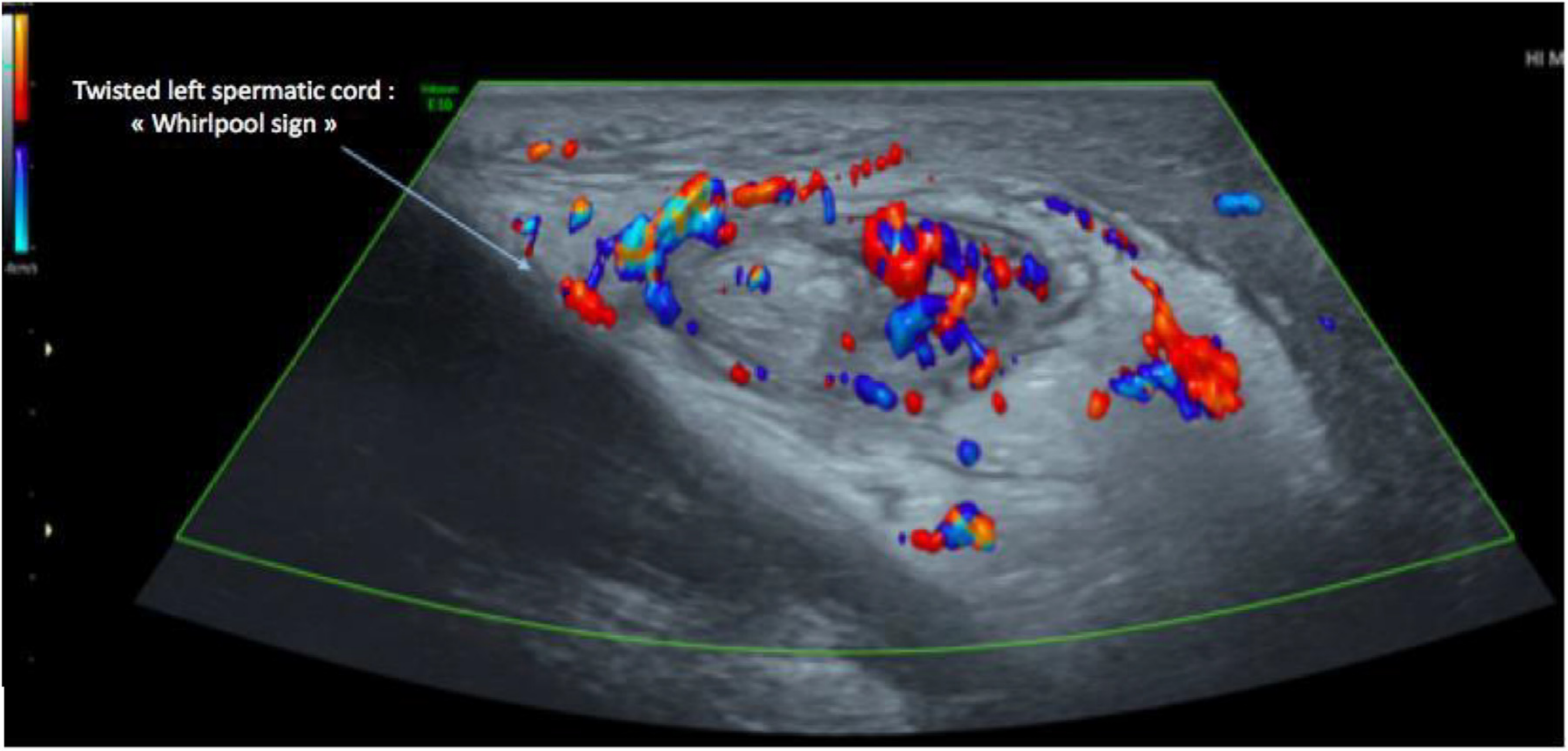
Study of the left spermatic cord in Doppler mode: presence of torsion with an image of a “Whirlpool sign” turn.
